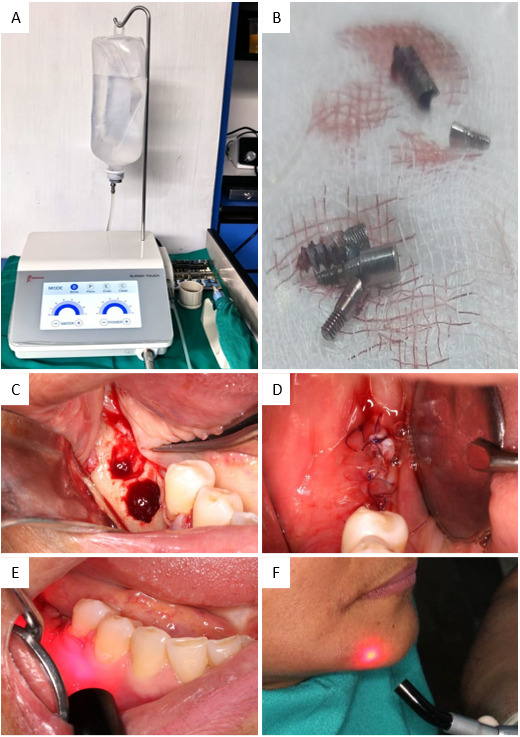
A) Dispositivo piezoeléctrico UDS-P Piezo Ultrasonic Scaler®. B) Implantes removidos quirúrgicamente. C) Fotografía intraoral posterior a la explantación múltiple. D) Cierre de tejidos blandos a través de la sutura en la zona quirúrgica. E) Aplicación de terapia láser intraoral con una longitud de onda de 650 nm. F) Aplicación de terapia láser extraoral con una longitud de onda de 976 nm.
